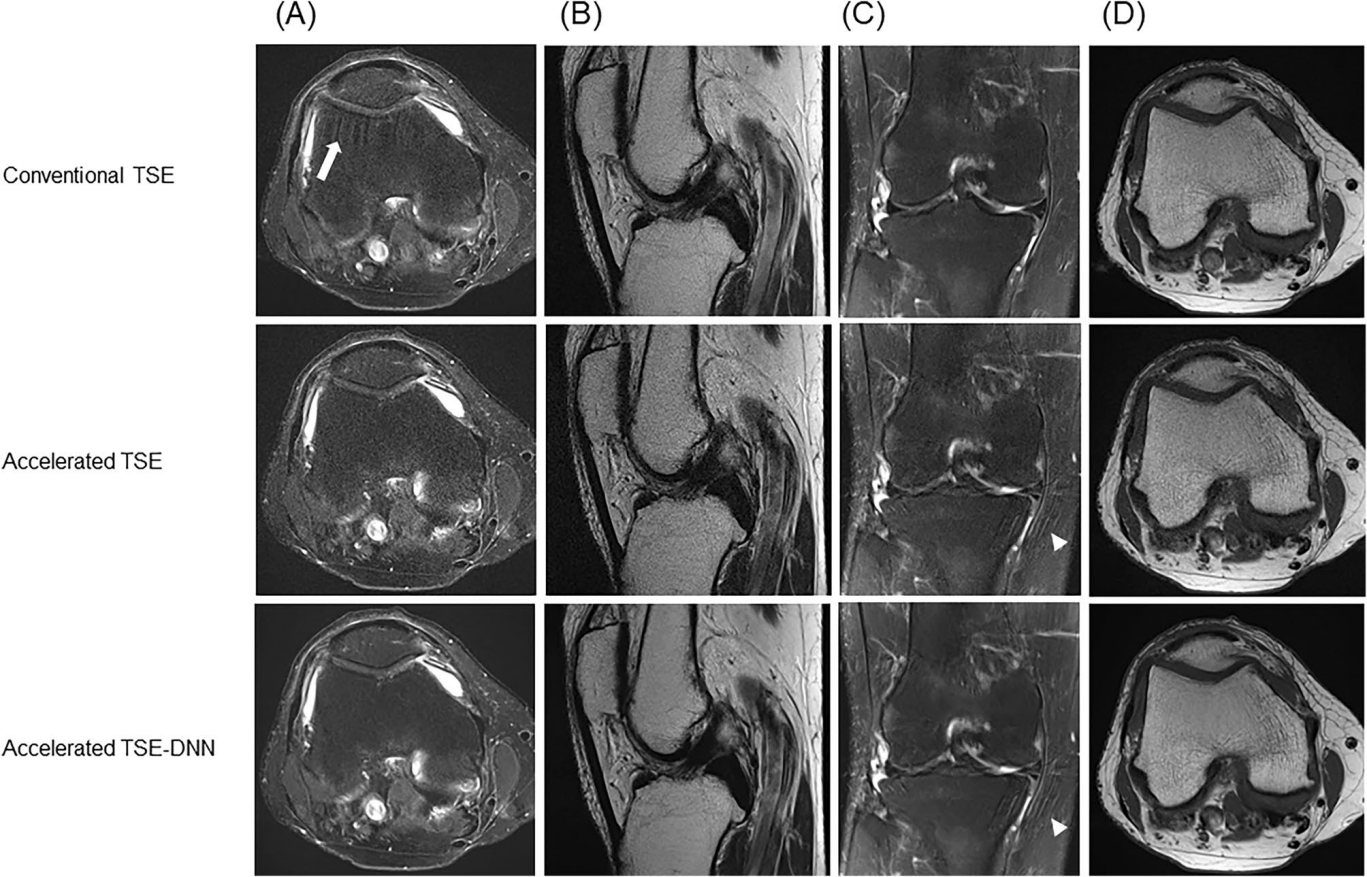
Conventional and reconstructed images of accelerated sequences: a 64-year-old woman with knee pain (A–D). Motion-related artifacts in conventional FSE (A upper, arrow) is not seen on accelerated image (A middle), and the image is enhanced on FSE-DNN image (A lower). Overall image quality is comparable in both conventional FSE and FSE-DNN images (B and D). However, parallel imaging artifacts cannot be completely removed in FSE-DNN image (C middle and lower, arrowheads). The first row represents conventional FSE images, the second row represents accelerated FSE sequences, and the third row represents DNN-reconstructed images of FSE-DNN. Each column represents the images reconstructed by axial fat-saturated T2-weighted image, sagittal T2-weighted image, coronal fat-saturated T2-weighted image, and axial T1-weighted image.
